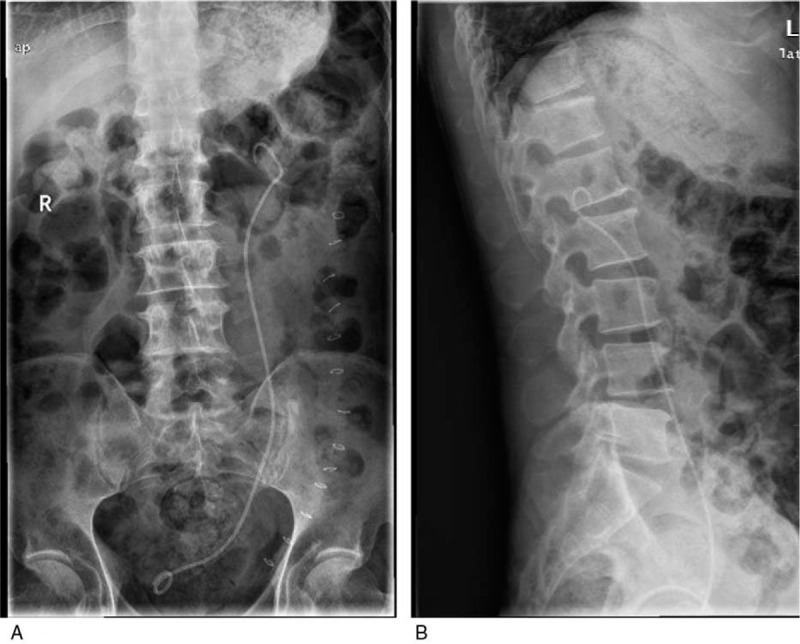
Post-operative x-ray radiograph showing the normal position of the double J stent.
